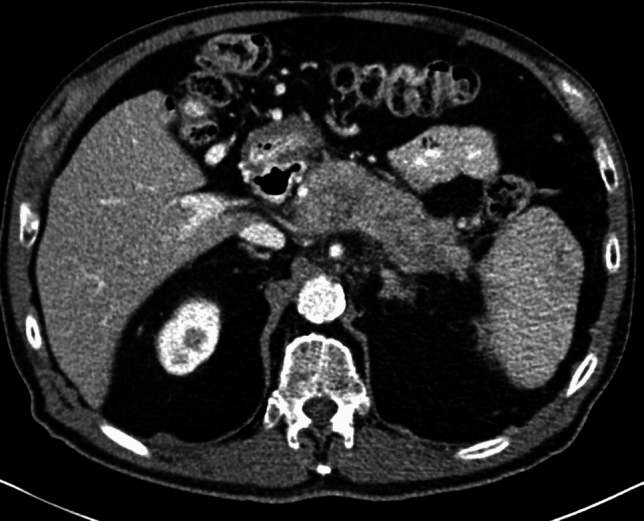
CT demonstrating hypoenhancing mass throughout the body and tail of the pancreas done at time of diagnosis of pancreatic cancer
